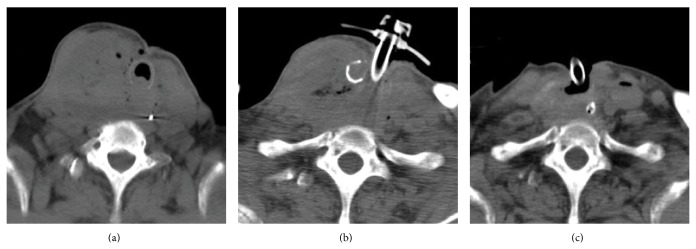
ATC in a 75-year-old woman. The cervical mass was inseparable from the strap muscles, tracheal wall, and carotid sheath. (a) Computed tomography scan showing a huge mass in the right thyroid robe on admission, (b) after surgical resection of a part of tumor and tracheotomy, and (c) after radiotherapy and seven cycles of chemotherapy.
